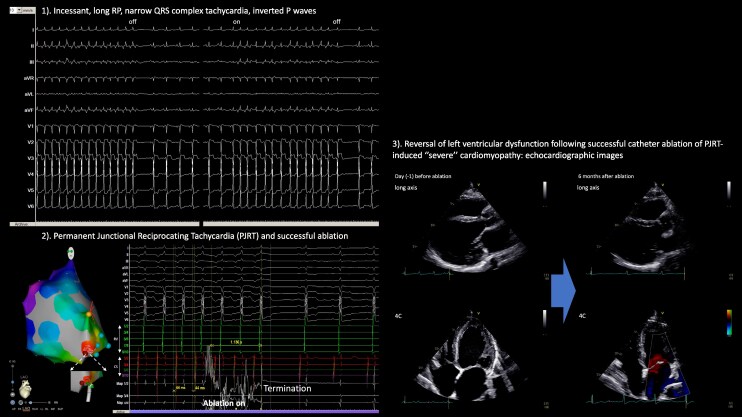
Graphic summary—severe cardiomyopathy due to permanent junctional reciprocating tachycardia: recovery after catheter ablation? (1). Incessant, long RP, narrow QRS complex tachycardia, inverted P waves. (2) Permanent junctional reciprocating tachycardia and successful ablation. (3) Reversal of left ventricular dysfunction following successful catheter ablation of PJRT-induced ‘severe’ cardiomyopathy: echocardiographic images.
